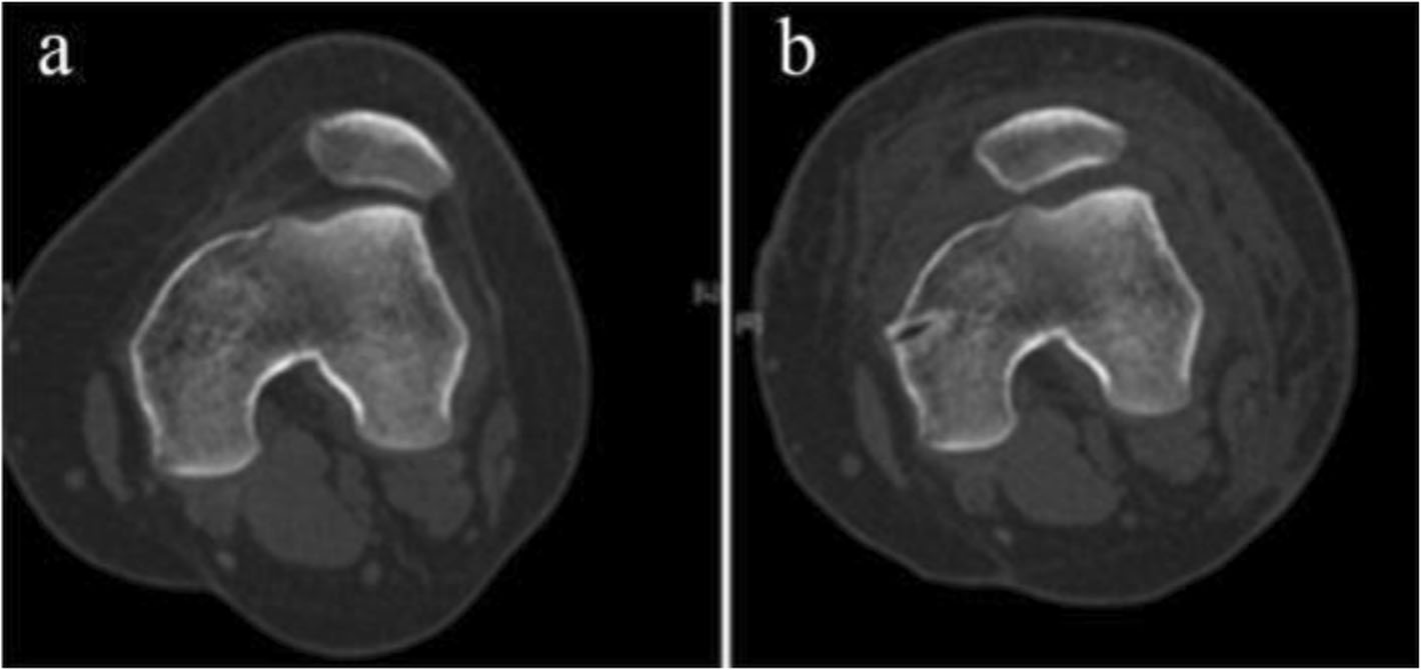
Comparison of preoperative (a) and postoperative (b) CT scans demonstrating restoration of patellofemoral tracking
